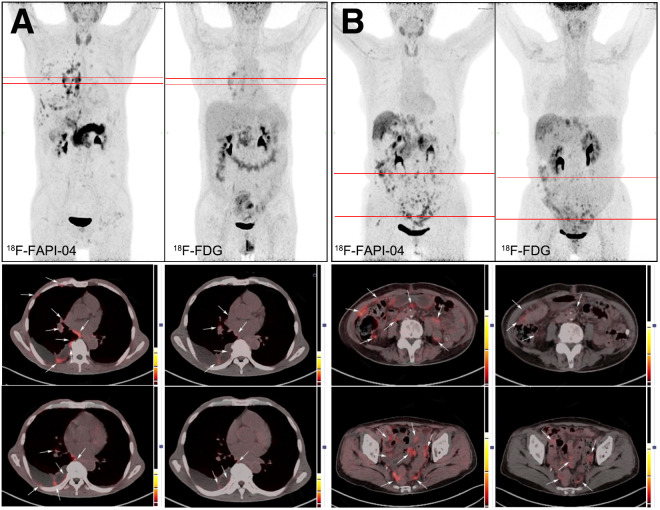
Typical PET (top) and PET/CT (middle and bottom) images showing pleural (A) and peritoneal (B) metastasis obtained with 2 tracers. Axial PET/CT images correspond to red lines in coronal PET images. Lesion is marked by arrows.
